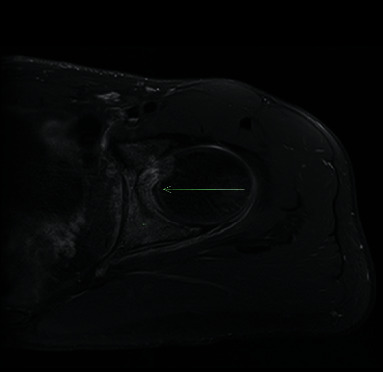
MRI contrast-enhanced T1 fat-saturated axial image demonstrating enhancing bone edema (^*∗*^) and medial synovial thickening with enhancement (arrow).
